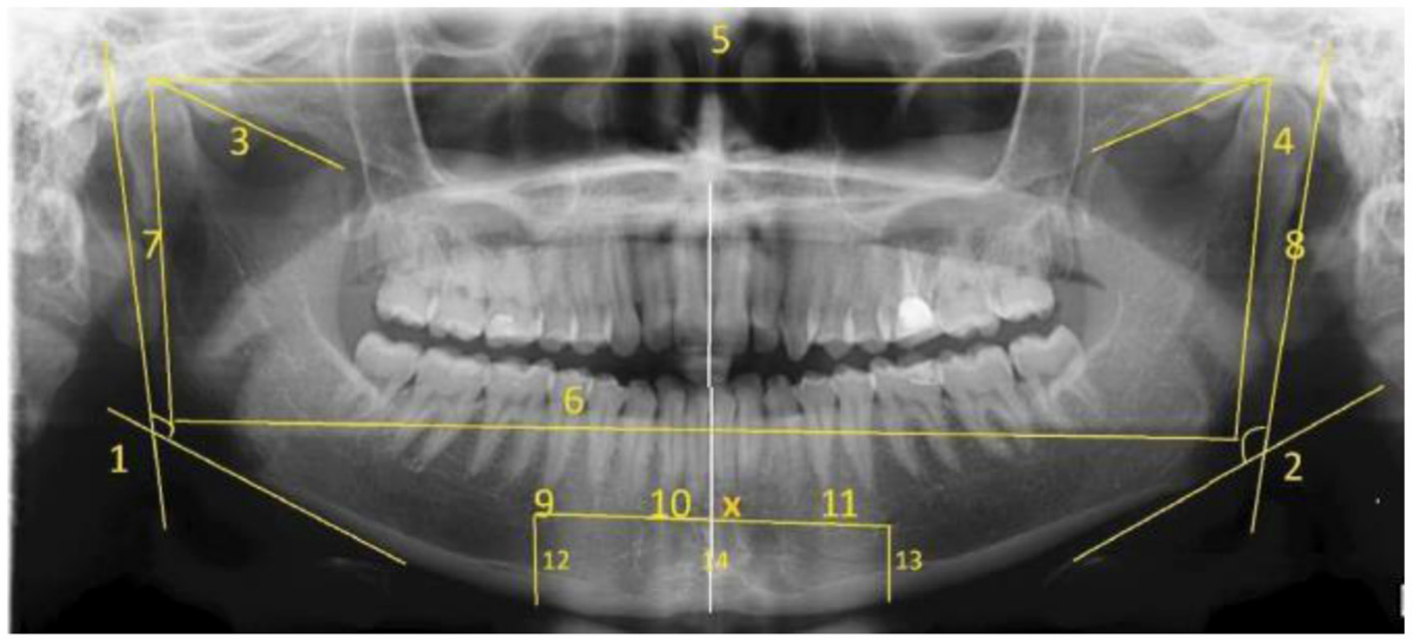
Measurement of morphometric parameters marked on panoramic radiographs (Ortiz et al., 2020). R, Right; L, Left; AMD, right mandibular angle; C, Condyle; Co, Coronary process; Go, Gonys; FM, Mental Foramen; PSM, Medium Saginal Plane; Me, Mento; BMD, Mandible Base; X Me, Me Intersection Point; PSM, Me.
